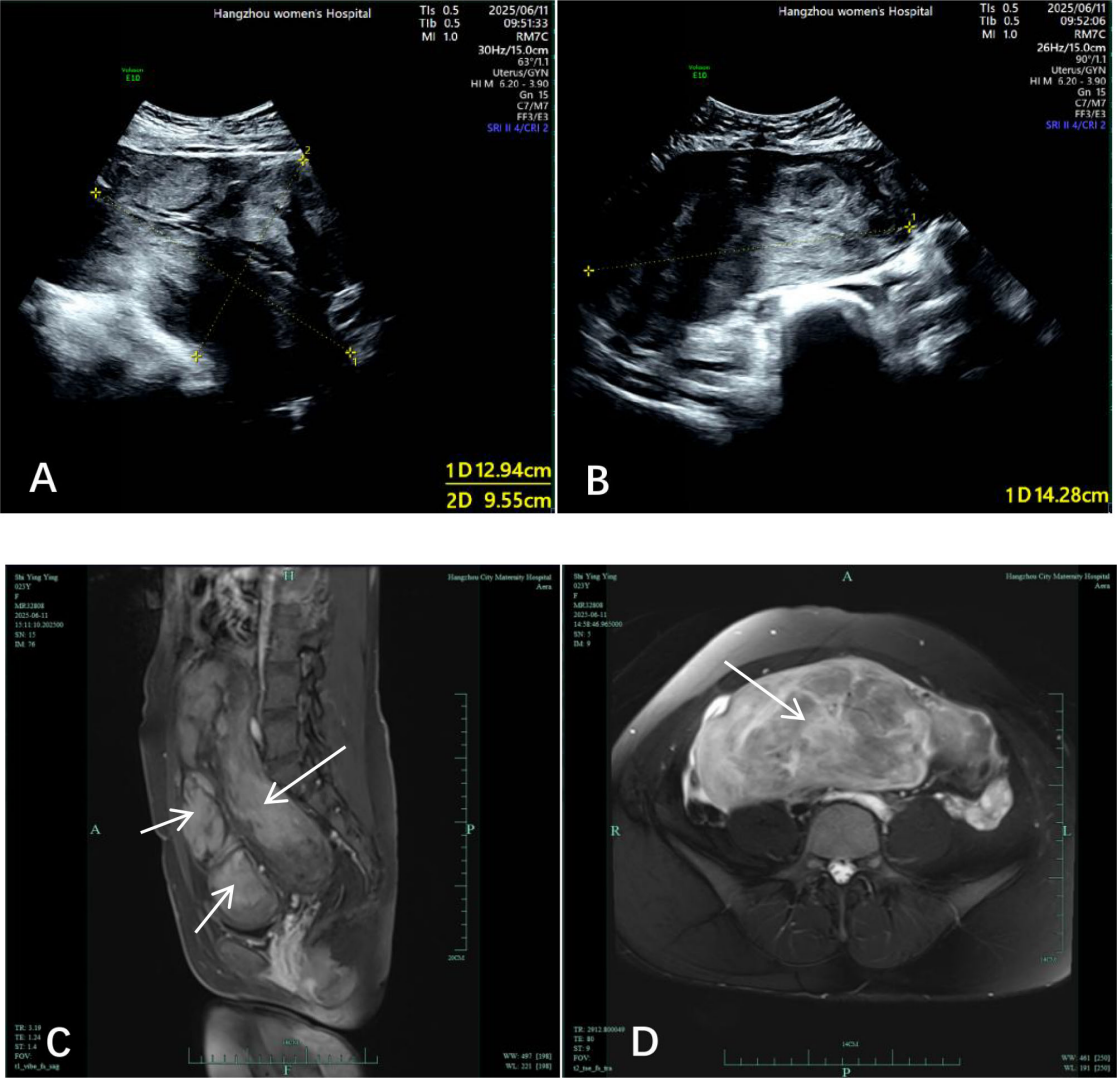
Preoperative transvaginal gynecological ultrasound of the patient revealed a massive abdominopelvic mass, which was considered to be of uterine origin with undetermined nature (A, B). Preoperative MRI revealed a massive mass shadow in the abdominopelvic cavity. On T1-weighted images (T1WI), the arrows indicate that the mass has relatively clear boundaries and exhibits lobulated margins (C) on T2-weighted images (T2WI), the arrows show that the mass fills the entire abdominopelvic cavity, adheres closely to the pelvic wall, and compresses the surrounding organs (D).
